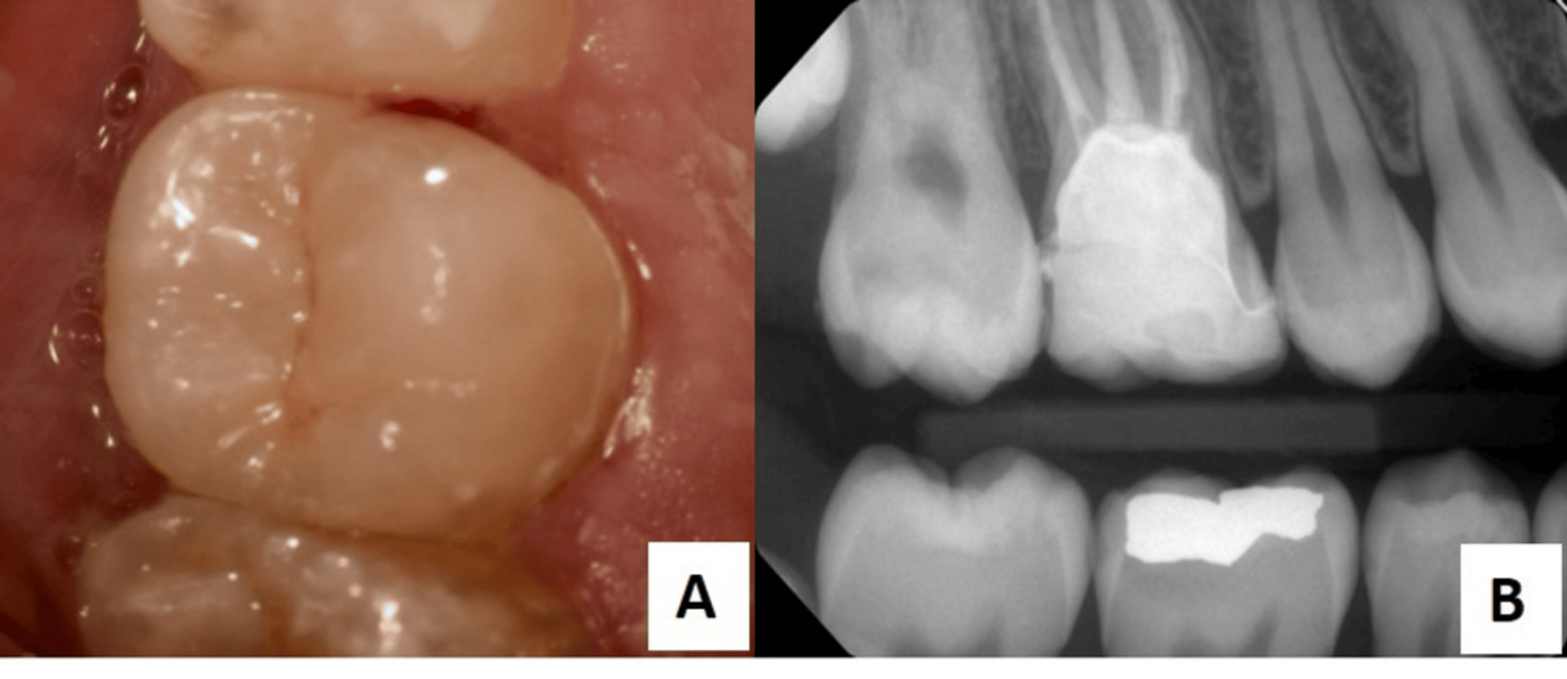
(A) Post-cementation intraoral photo; (B) Post-cementation bitewing radiograph.
